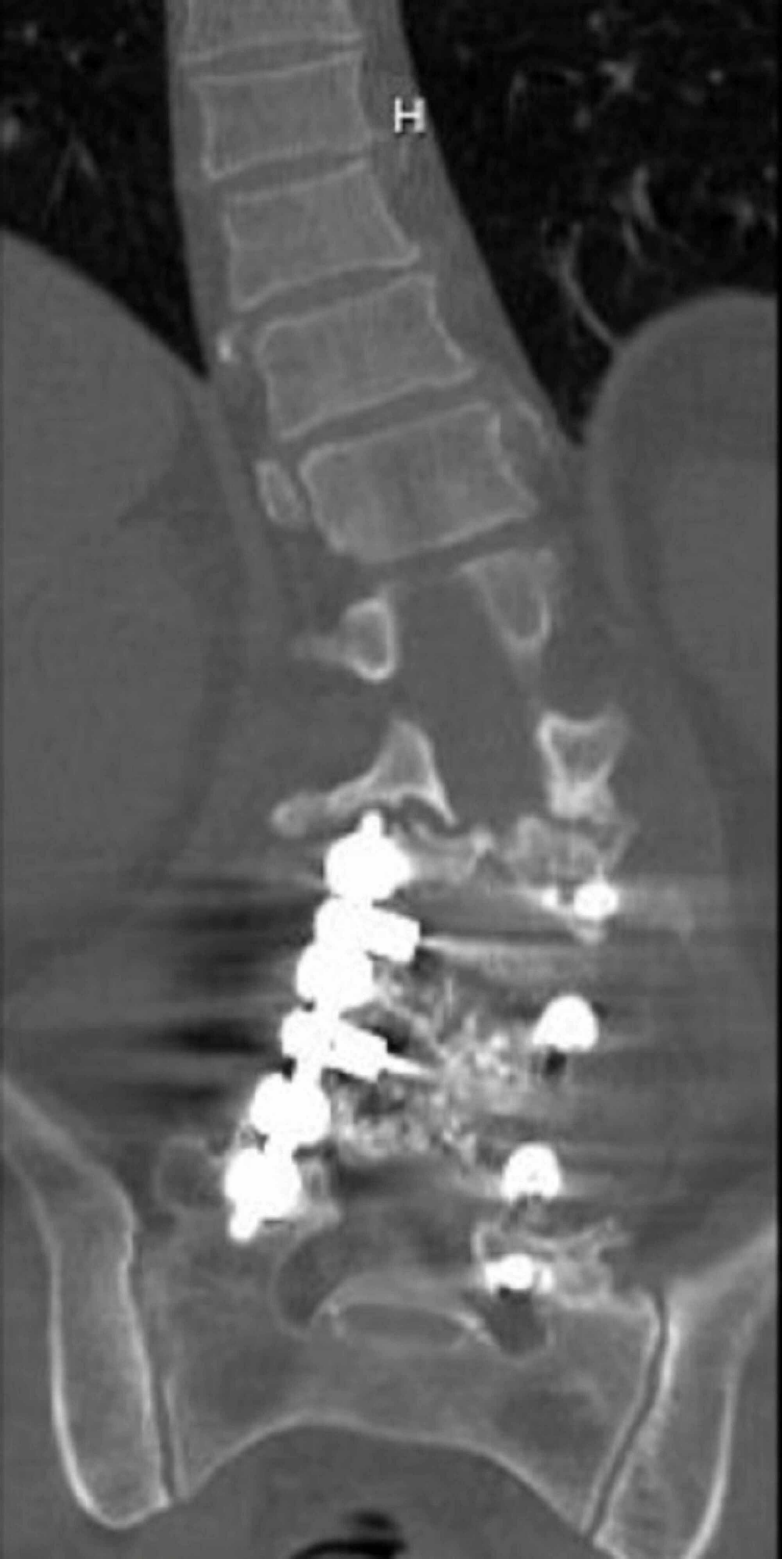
Preoperative CT of the lumbar spine, coronal view, displays the curvature of the patient's lumbar spine and identifies the existing hardware from prior surgery. CT, computed tomography.
